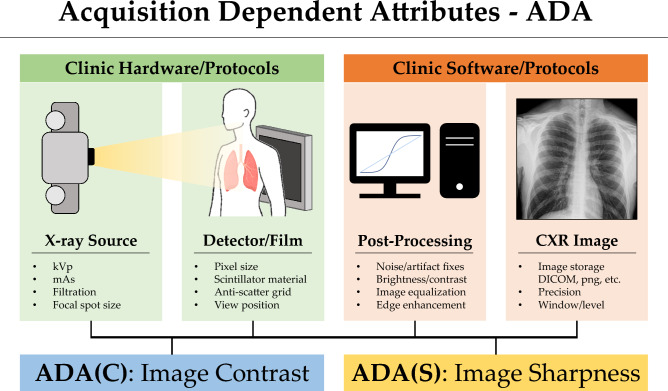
Acquisition dependent attributes (ADA) in chest x-ray images.
